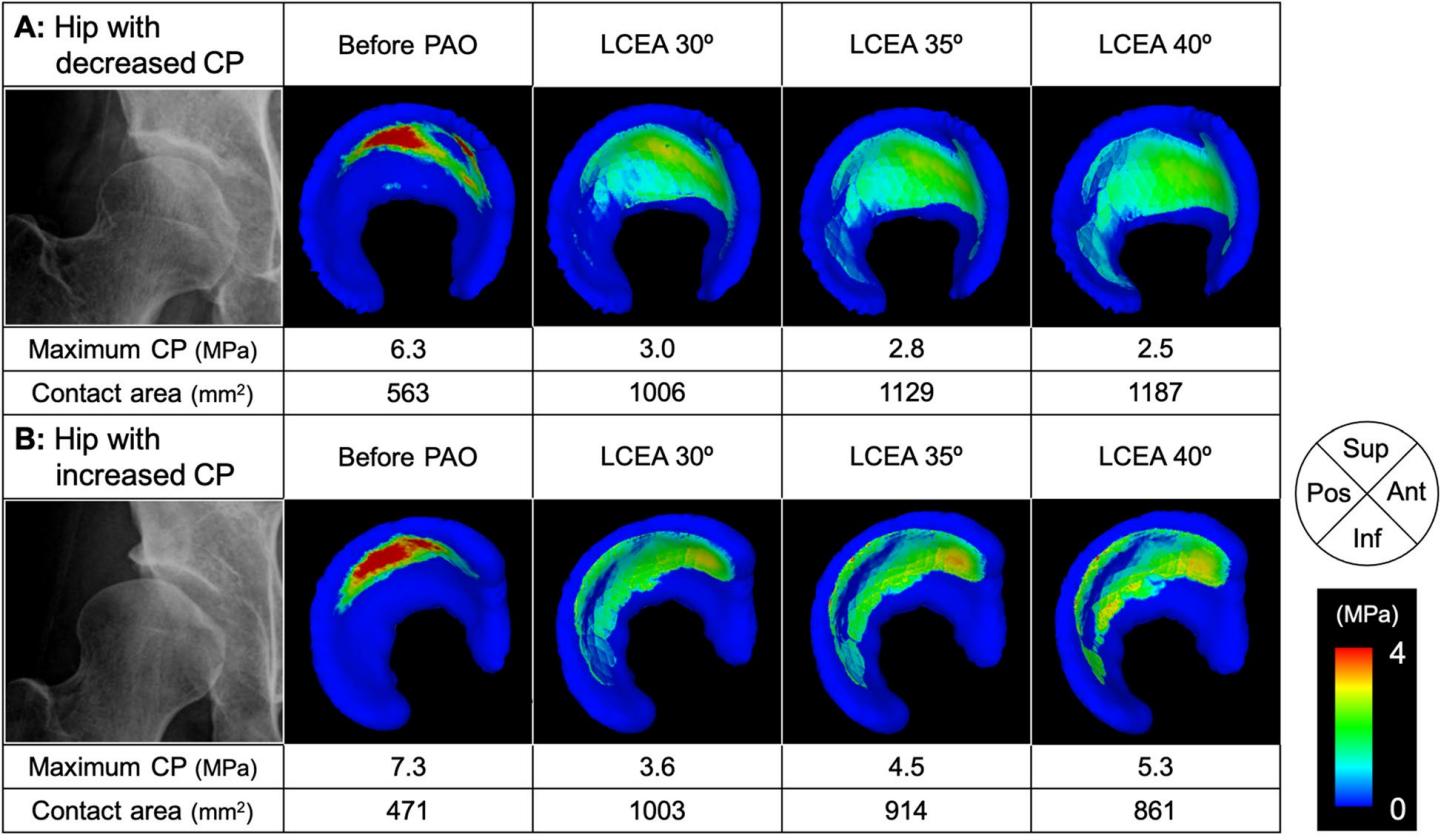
Preoperative anteroposterior pelvic radiographs and distributions of joint contact pressure (CP) on the acetabular cartilage before and after virtual periacetabular osteotomy (PAO) in two representative cases with hip dysplasia (right dysplastic hips). A In one case, the maximum CP decreased by increasing the lateral center-edge angle (LCEA) from 30° to 40°, while (B) in the other case, the maximum CP increased. Case B had larger extrusion index (47.2 vs. 27.6) and roundness index (53.4 vs. 52.1) than case A. The reference locations of the acetabular cartilage at the standing position are denoted as anterior (Ant), superior (Sup), posterior (Pos), and inferior (Inf)
